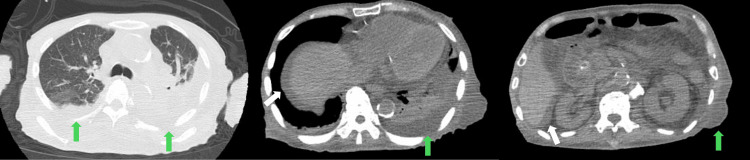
Chest and abdominal computed tomography (CT) on admission. CT showed bilateral pleural effusion (the green arrow on the left and middle panels), ascites (the white arrow on the middle and right panels), and subcutaneous edema (the green arrow on the right panel).
